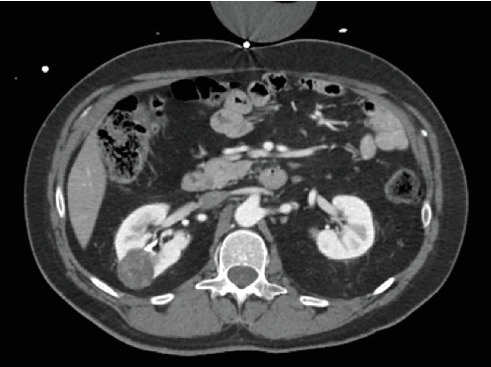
A CT scan of the abdomen and pelvis with IV contrast shows a 3 cm heterogeneous lesion in the right kidney indicative of a complex cystic mass.
